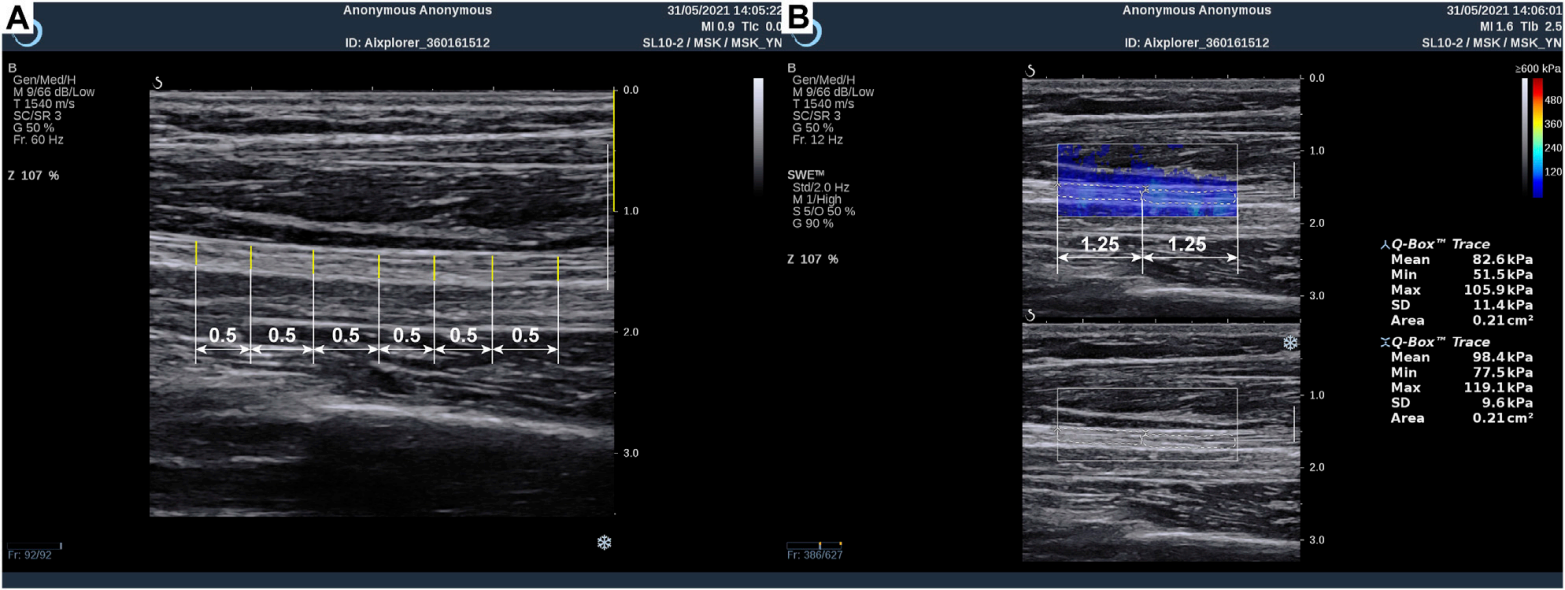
Method of median nerve ultrasonic measurement: (A) thickness measurement and (B) Young’s modulus measurement.
